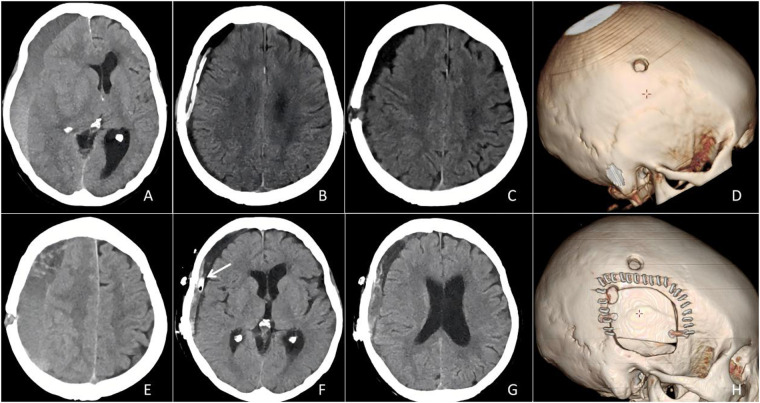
Preoperative and postoperative cranial CT scans in a representative patient. (A) Initial preoperative chronic subdural hematoma. (B) Postoperative day 2 CT after first burr-hole drainage. (C) Pre-discharge CT after first surgery. (D) Burr-hole site from the first procedure. (E) Hematoma recurrence at 2 months postoperatively. (F) Postoperative day 1 CT after modified craniotomy (arrow: drainage tube). (G) Cranial CT obtained four days after the second surgery, prior to discharge; the increased density beneath the bone flap corresponds to the applied temporalis muscle. (H) Bone flap replacement during the second surgery; the bony defect beneath the flap was created intraoperatively using a milling cutter to allow passage of the temporalis muscle.
